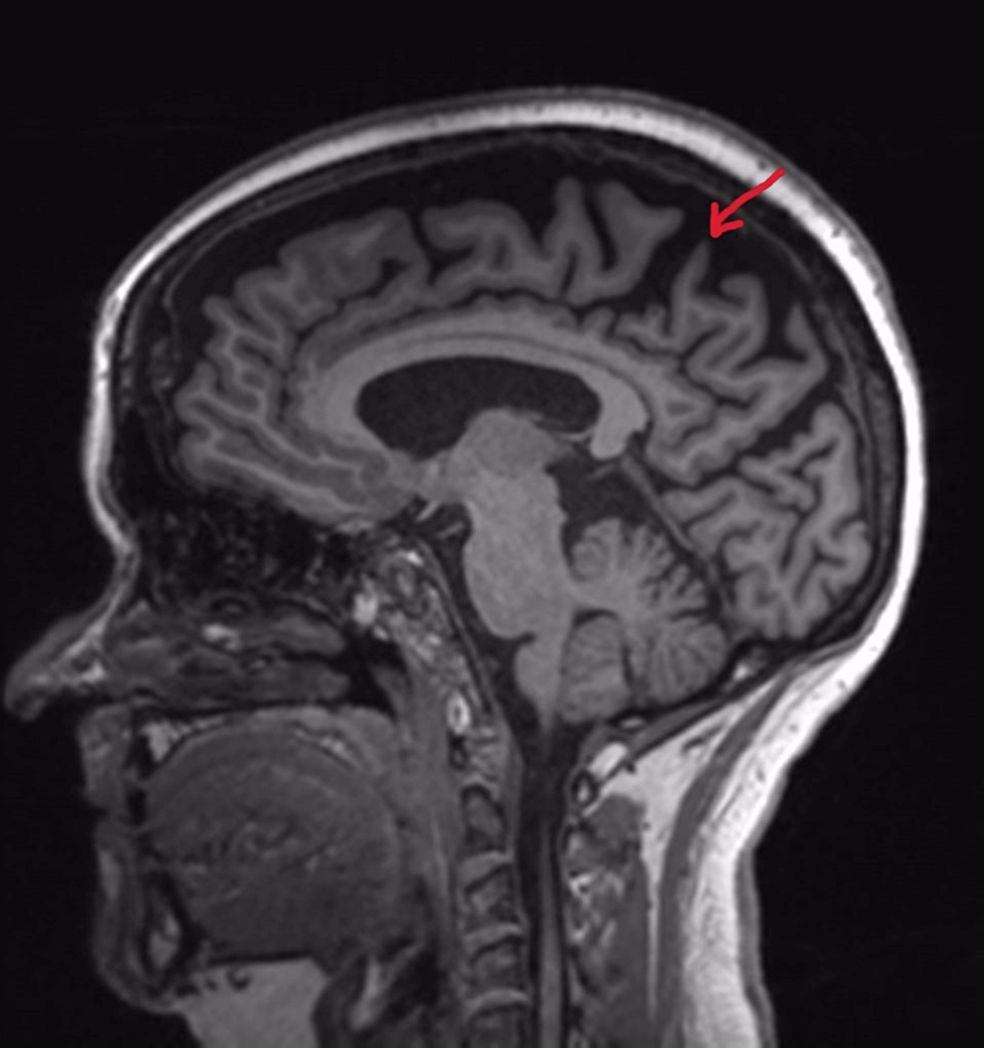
MRI Brain Sagittal T1 indicating generalised cortical atrophy with prominent atrophy in parietal and occipital regions
